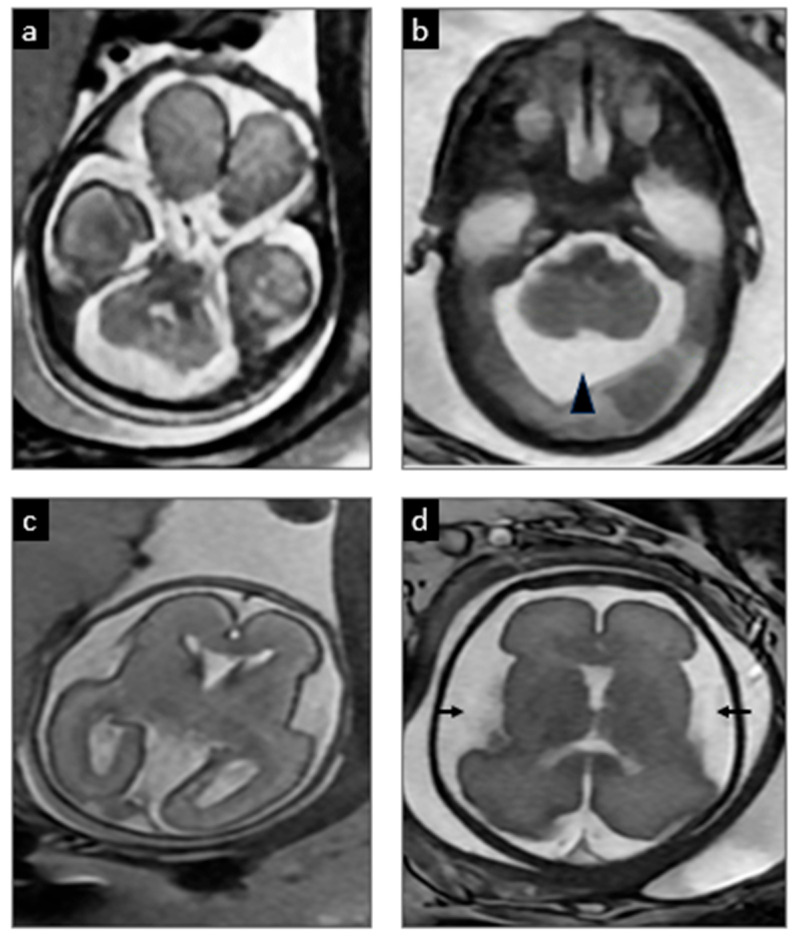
Serial prenatal MRIs. At 22 weeks 5 days gestation (a,c), the initial MRI showed normal development. However, at 28 weeks 5 days (b,d), there was evidence of a developing mega cisterna magna (arrowhead) and increased extra-axial spaces (arrows), which are common in MoCD and suggested emerging injury.
